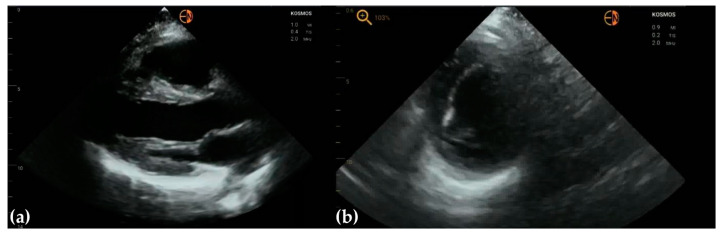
(a) Parasternal long axis view in a 57-year-old man presenting with aggravated chest angina shows an appropriate delineation of endocardial borders, allowing optimal measurement of heart structures and evaluation of LV motion; (b) parasternal short axis view shows obscuring of the LV antero-lateral wall in a 72-year-old man who was a smoker and presented with chest angina, probably because of lung reverberation effect; this was not noticed with the high-end device.
